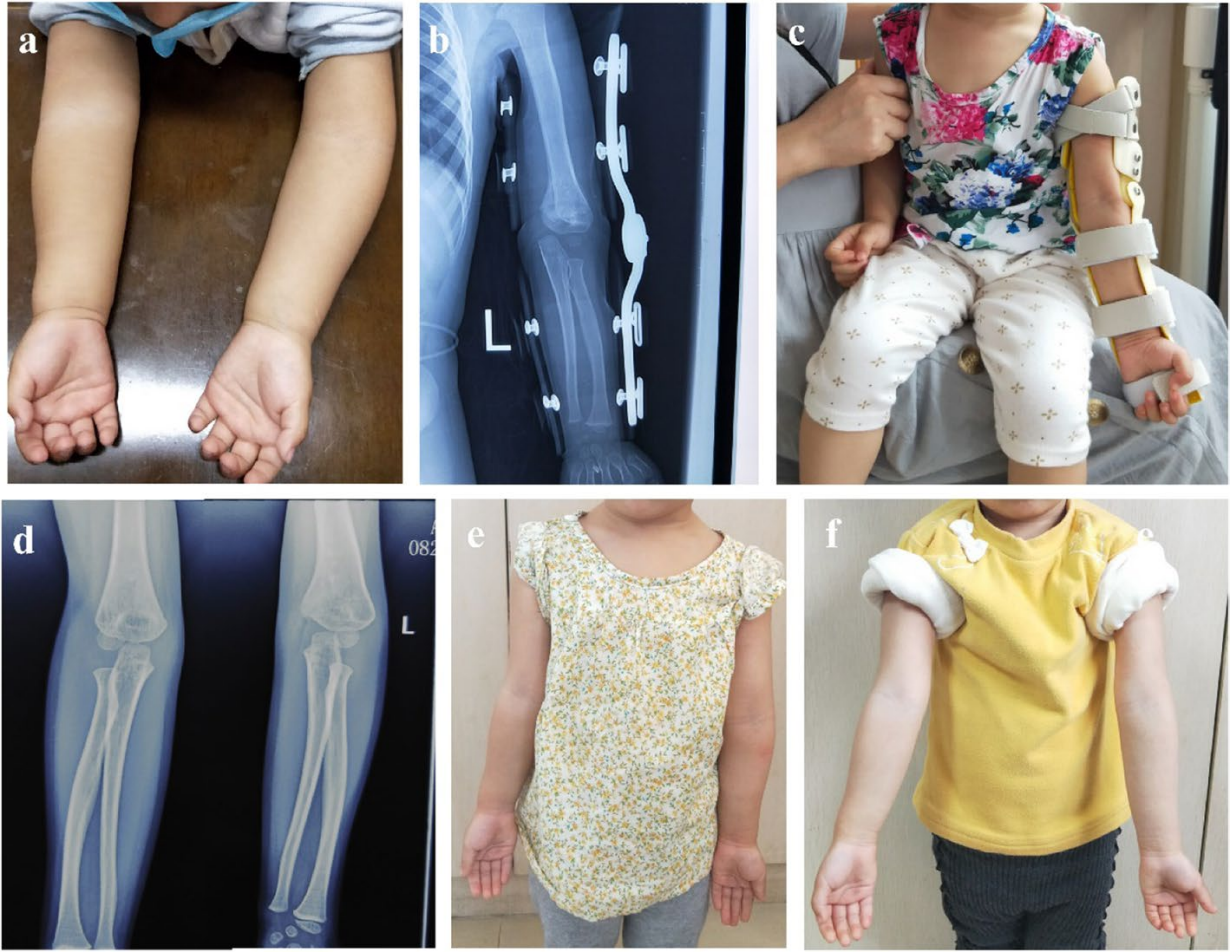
Case 2: a Pre-treatment clinical appearance of left cubitus varus deformity. b,c X-ray and clinical appearance after wearing the functional brace, respectively. d Post-treatment radiograph of bilateral upper limb at 1 year. e,f Clinical photographs of bilateral upper limb at 1 year and last follow up, respectively
